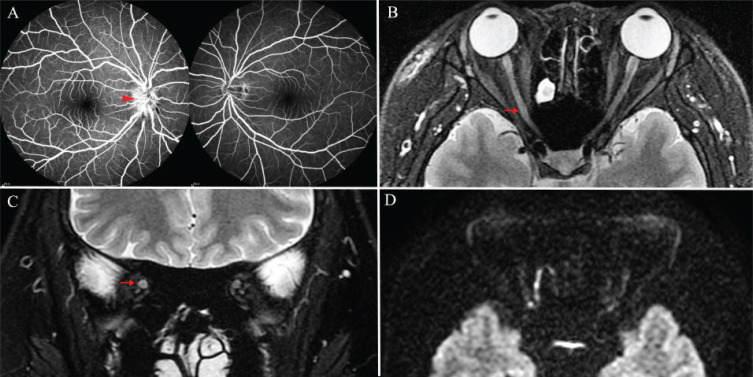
A 23-year-old man with IDON. A: FA: Right disc edema with fluorescein leakage (red arrowhead). B: Axial; C: Coronal T2-weighted MRI; Discrete T2-weighted hyperintense signal in the right optic nerve (red arrow). D: DWI: No high signal intensity in either optic nerve.
